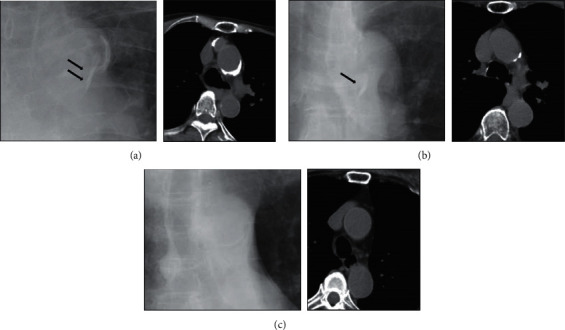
The classification of the Aortic Arch Tail Sign. The prominent calcification line confirmed by plain film and CT imaging was classified as +2. A line which was ambiguous with plain film imaging but was confirmed by CT was classified as +1. Images classified as 0 showed no calcification. (a) +2. (b) +1. (c) 0.
